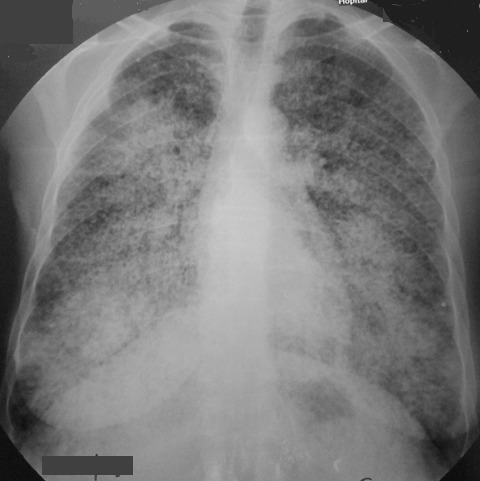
Radiographie thoracique de face: opacité micronodulaire disséminée, confluente dans les deux champs pulmonaires
